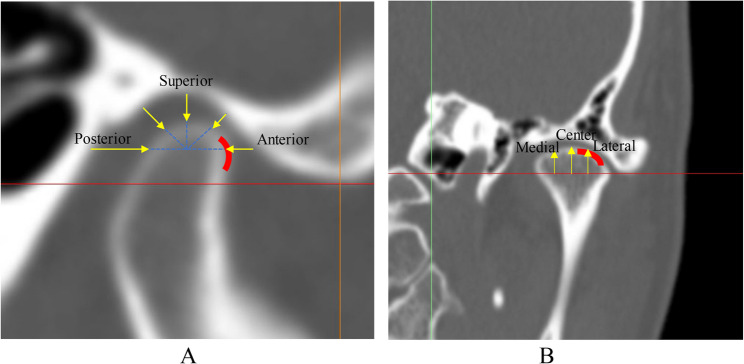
Condylar surface CT values. Red lines indicate regions with higher CT values. A Sagittal plane: higher CT values in Class II than Class III; anterior region > posterior region; postoperative (1 year) > preoperative. B Coronal plane: higher CT values in Class II than Class III; lateral region > medial region; postoperative (1 year) > preoperative
